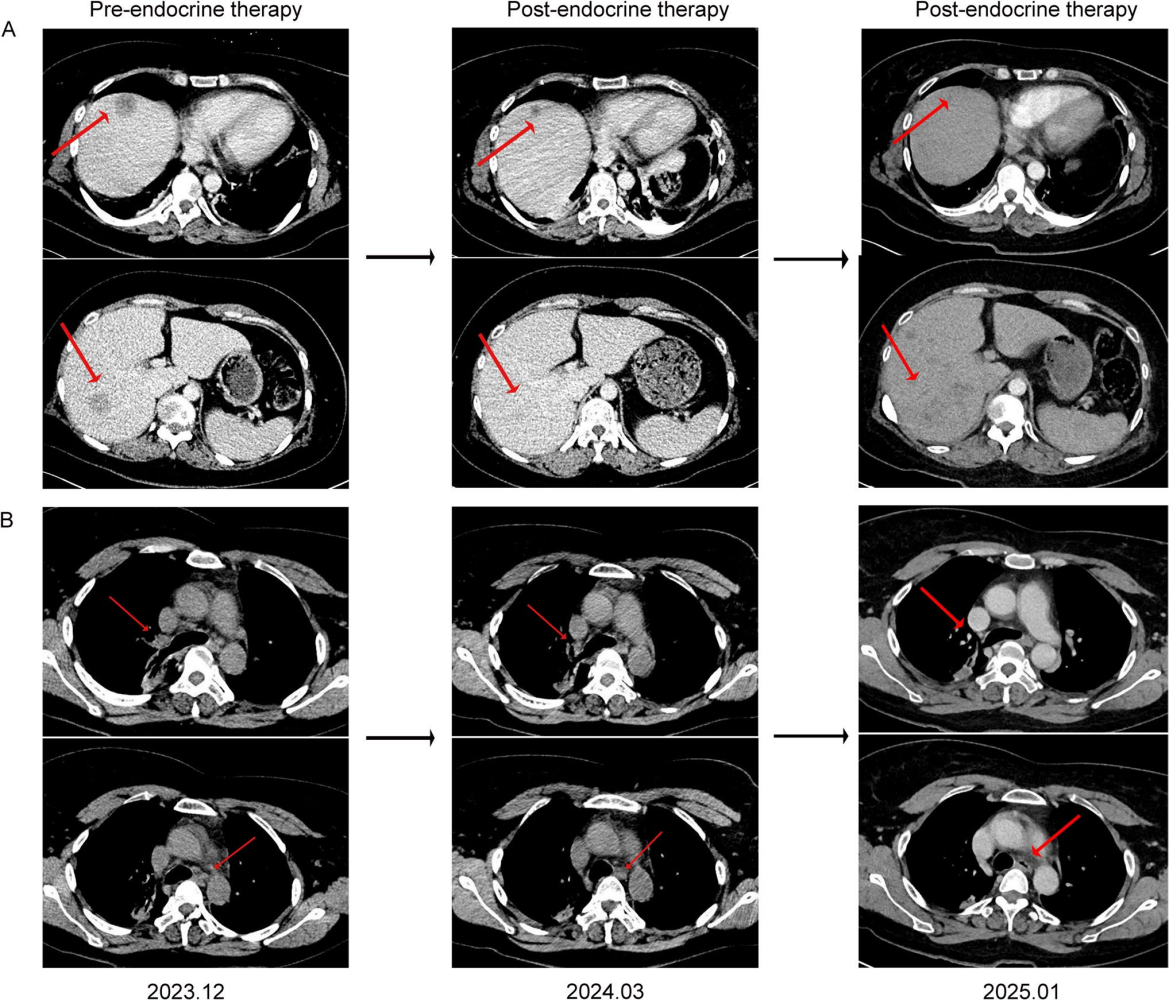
A Computed tomography scans of metastatic hepatic nodules at baseline (left) and after endocrine therapy (right). B Computed tomography scans of metastatic mediastinal lymph nodes at baseline (left) and after endocrine therapy (right)
